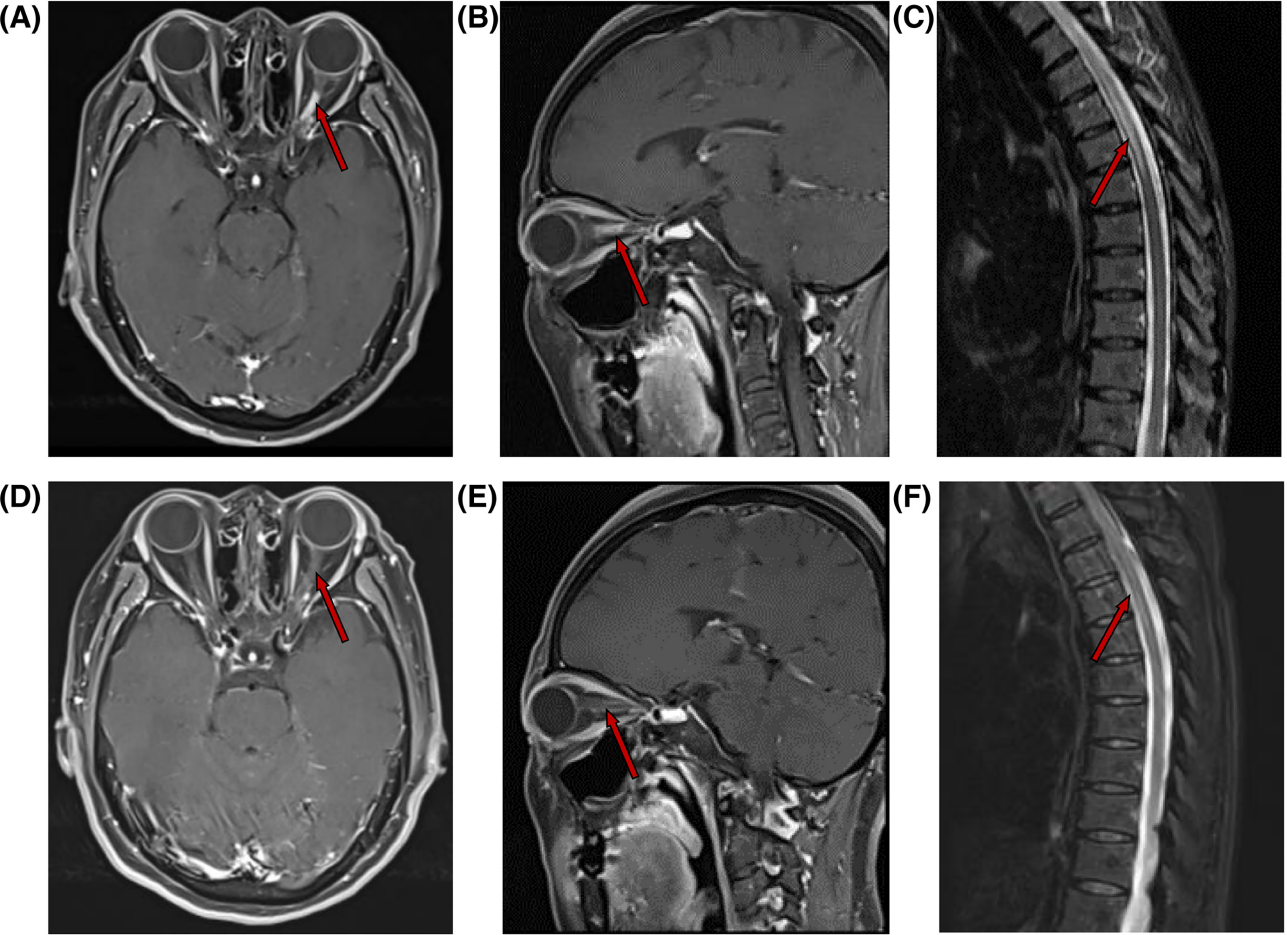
(A, B) Patient 2 hospitalized due to blindness of left eye for more than 1 week and narrowed vision of right eye for 4 days. Figure (A) shows enhanced signal of left optic nerve in gadolinium enhanced at baseline T1WI. Figure (B) shows gadolinium enhanced T1WI enhancement in sagittal position of left eye at baseline. (C) Thoracic MRI of Patient 1 at baseline. There was a high signal lesion of T2WI in the thoracic medulla at T3–T7 level. (D, E) Patient 2 was reexamined with MRI after 48 weeks of treatment. Enhanced signal of left optic nerve in gadolinium enhanced T1WI was improved. An orbital‐like enhancement was observed in the optic nerve sheath in gadolinium enhanced T1WI. The left optic nerve sheath was thickened, considering the chronic change of optic myelitis. (F) Thoracic MRI of Patient 1 at week 48. The high signal lesion of T2WI in the thoracic medulla at baseline diminished after Telitacicept treatment
